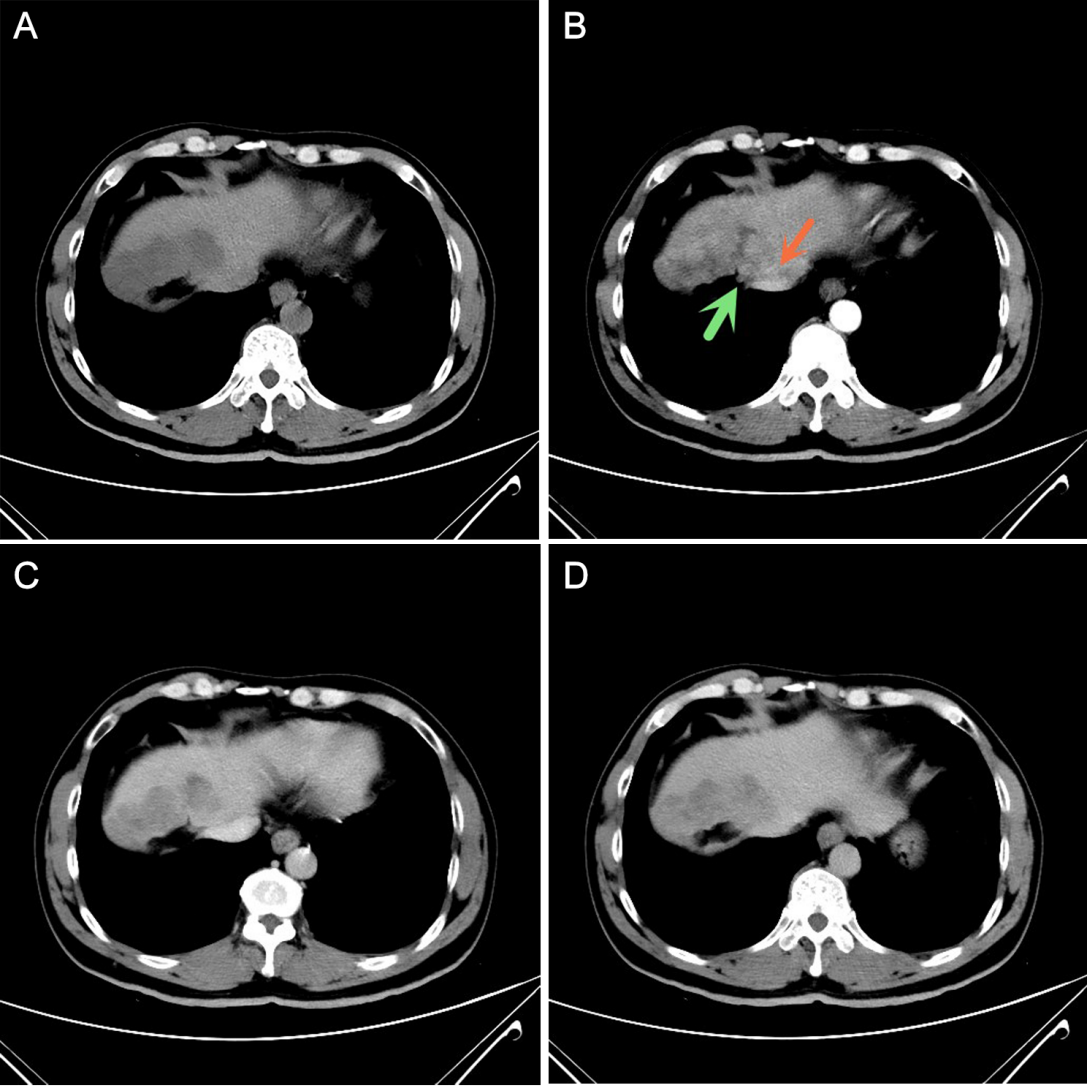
cHCC-ICC presenting with MVI in a 48-year-old man. (A) A plain CT scan showed an irregular low-density mass; (B-D) Early enhancement of the mass was observed during the arterial phase of CT enhancement, followed by rapid clearance of the contrast agent from the mass. Hepatic capsule retraction (green arrow) and peritumoral enhancement (red arrow) were observed
